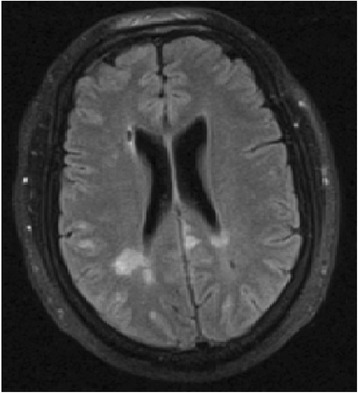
T1-weighted MRI scan showing cortical white matter and subcortical and deep periventricular small ischaemic lesions of old and new age
